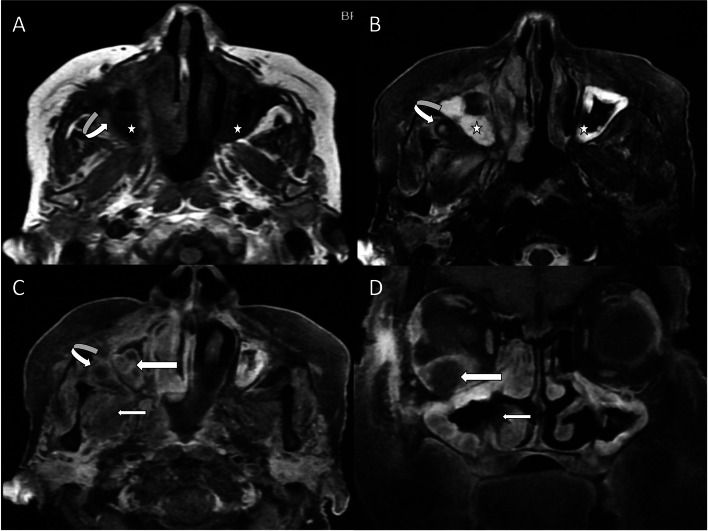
Sinu-nasal mucormycosis with extension into retroantral fat, pterygoid muscle, and orbit. Axial T1-weighted image (A) shows hypointense mucosal thickening of the walls of bilateral maxillary sinuses (white stars) with the erosion of posterior wall of right maxillary sinus (curved arrow). Axial T2-weighted fat-saturated image (B) shows a well-defined hypointense lesion in right retroantral region (curved arrow) with central hyperintensity. Mucosal thickening appears hyperintense (white stars). Axial contrast-enhanced fat-saturated T1-weighted image (C) shows peripheral enhancement of the sinus mucosa (straight long arrow) and right retroantral lesion (curved arrow). Note the involvement of the right pterygoid muscle (straight short arrow) which appears bulky and shows heterogeneous enhancement. Coronal contrast-enhanced fat-saturated T1-weighted image (D) shows a peripheral enhancing lesion in the right orbit with central non-enhancing area (straight long arrow) suggesting intraorbital abscess formation. Part of the right inferior turbinate (straight short arrow) shows no enhancement (the “Black turbinate sign”)
